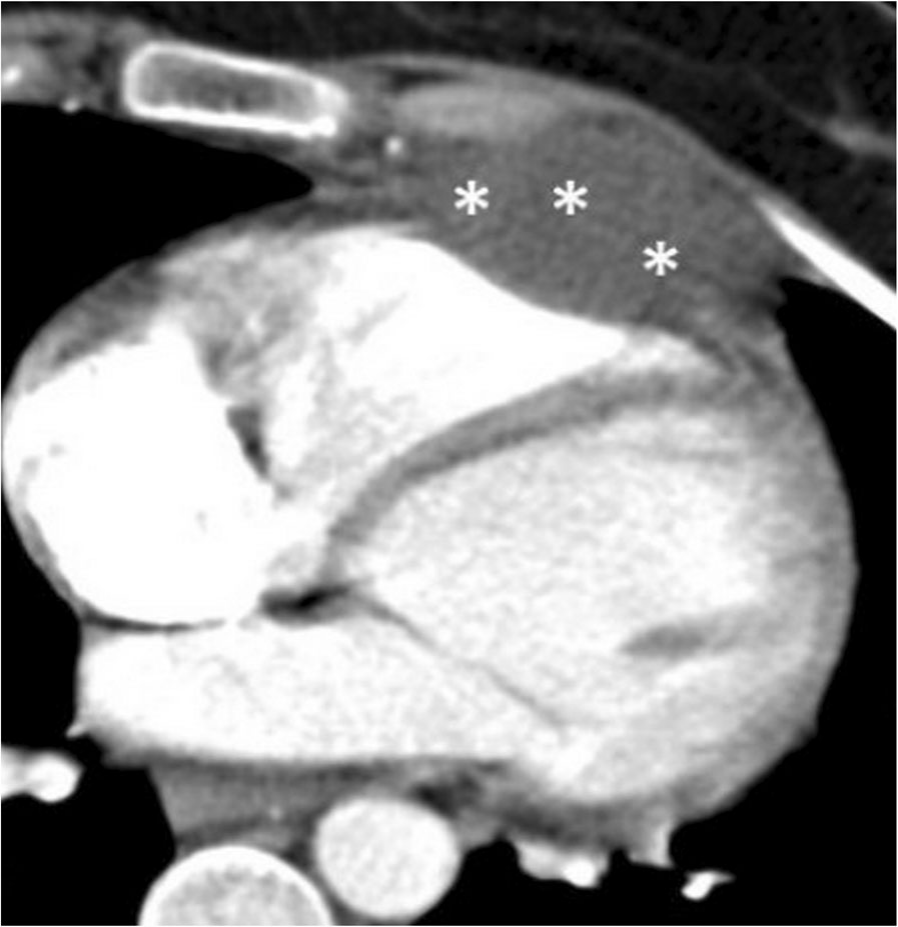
A 28-year-old woman with pathologically proven metastatic osteosarcoma. Axial contrast-enhanced CT image reveals a lobulated hypodense mass (asterisks) invading the anterior aspect of the right ventricle and pericardium
